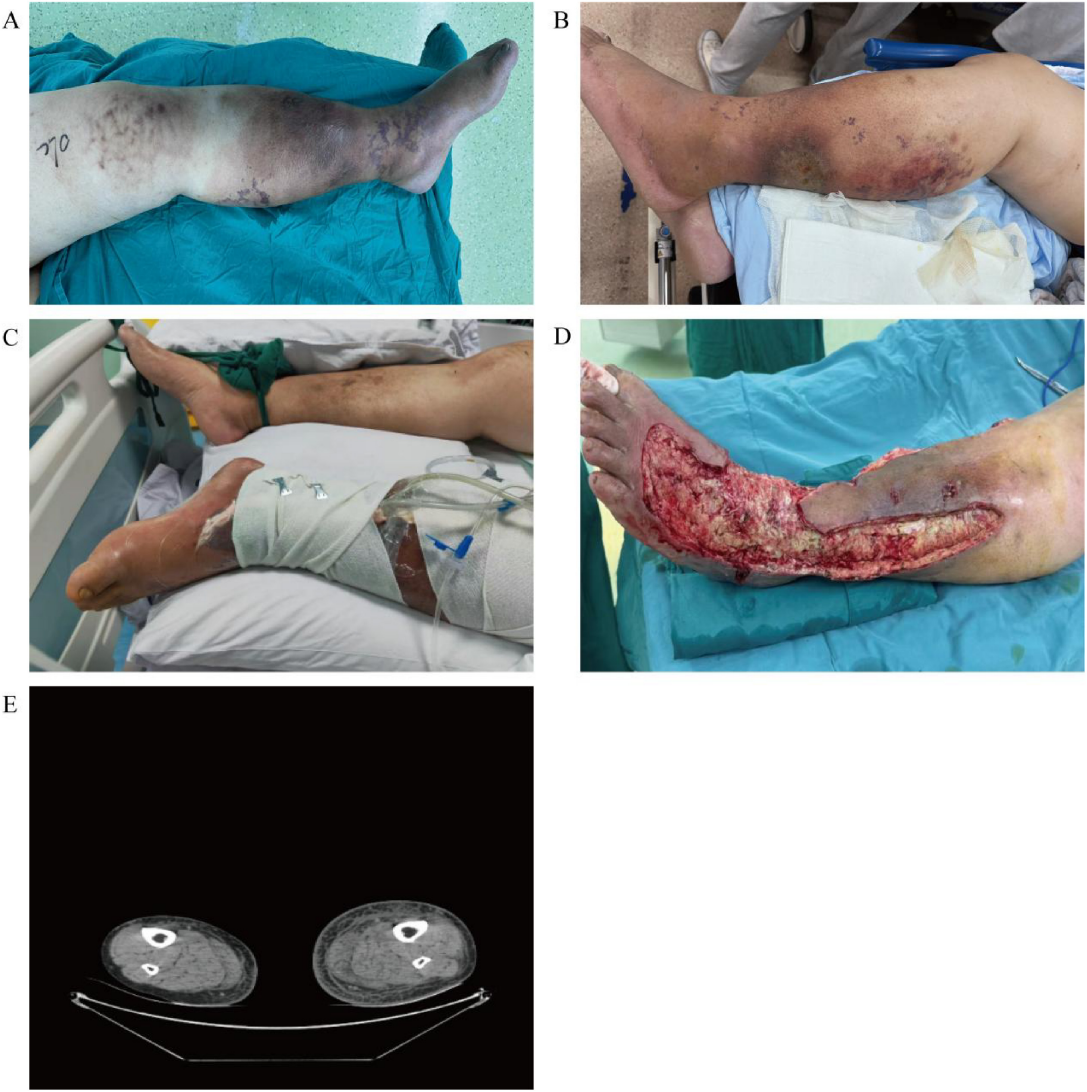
(A,B) On the day of admission, the patient’s left lower limb exhibited erythema and swelling; a small, exudative wound was visible on the posterior calf, and extreme compartment tension had led to the formation of tense bullae. (C) Post-operative photograph after the second procedure: VSD, escharotomy and decompressive fasciotomy of the lower-limb compartments. (D) Intra-operative view during the third procedure: pedicled flap transfer, escharotomy and VSD. Extensive skin necrosis extended from the mid-calf to the dorsum of the foot; bilateral relaxing incisions exposed posterior, dorsal, medial and lateral areas of eschar-covered necrosis, with underlying fascia and adipose tissue largely devitalised. (E) On the day of admission, CT revealed multifocal soft-tissue swelling and exudation involving the left knee, lower leg and ankle.
